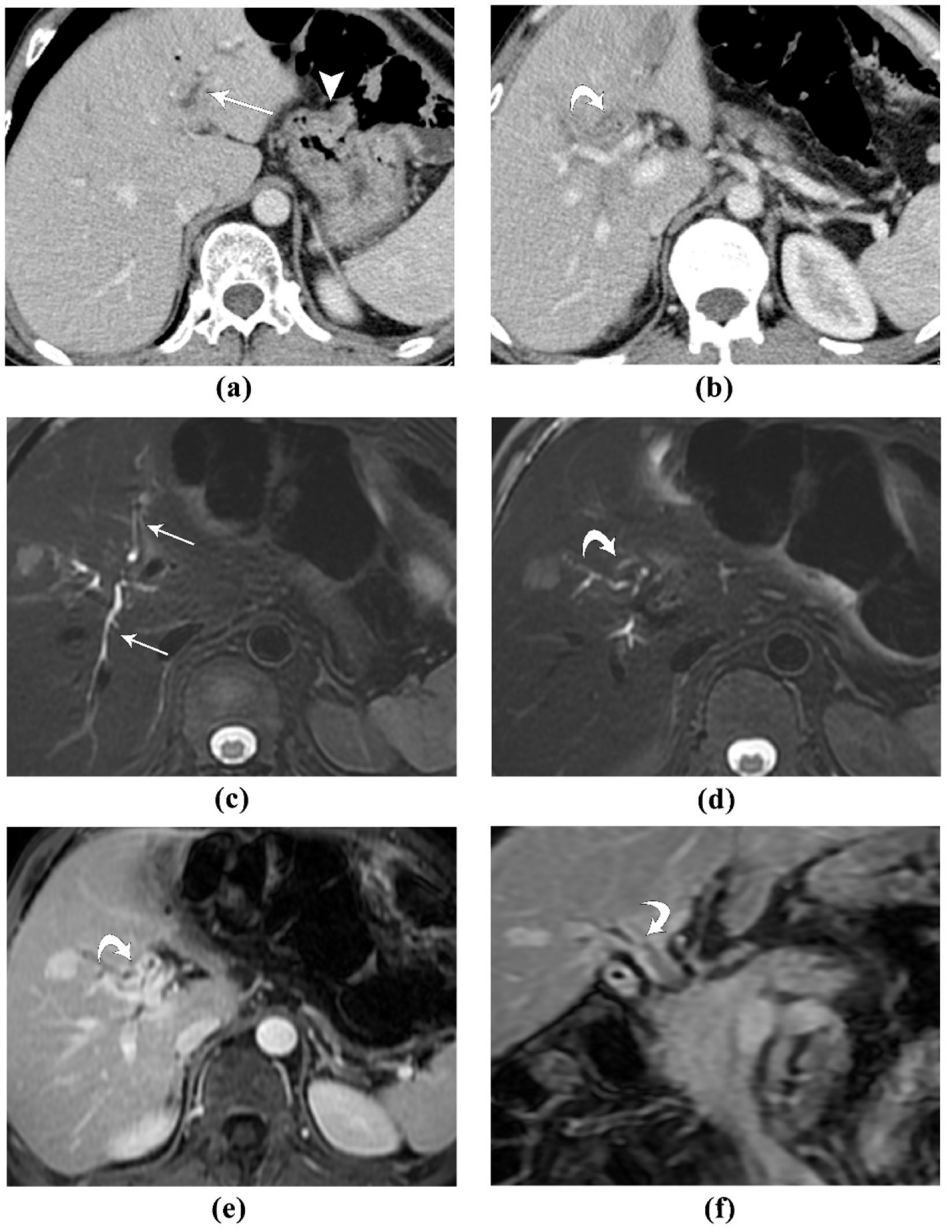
A 53-year-old male patient, who developed obstructive jaundice after 23 years of Bilroth II gastrectomy for peptic ulcer: (A, B) contrast-enhanced CT, portal venous phase images. The intra-hepatic bile ducts are dilated (*straight arrow*). The gastrojejunal anastomosis is seen (*arrowhead*). At the level of the bifurcation of left and right hepatic ducts, the bile duct wall is markedly thickened, with slight hyper-enhancement (*curved arrow*); (C, D) MR T2-weighted fat-saturated images. The intra-hepatic bile ducts are dilated (*straight arrow*), and the bile duct wall is thickened, which demonstrates intermediate to low T2 signal intensity; (E, F) contrast-enhanced MR, axial a coronal portal venous phase image. The hilar bile duct and the bifurcation of left and right hepatic duct demonstrated strong and homogeneous enhancement. This patient was diagnosed with IgG4-realted sclerosing cholangitis, which resolved after steroid therapy. Both contrast-enhanced CT and MRI depicts the location and extent of the lesion well, and correctly characterized it as benign.
